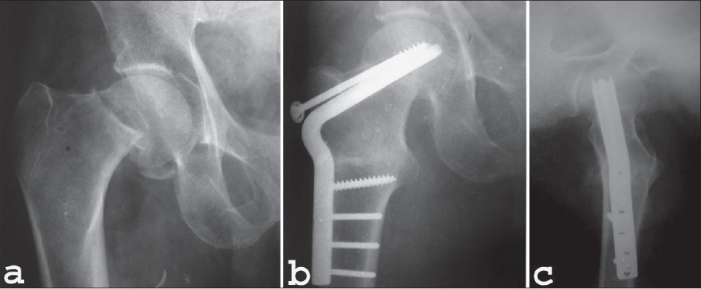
A: Antero-posterior radiograph (a) of 80 years male shows Garden stage IV and Pauwels type III femoral neck fracture. Anteroposterior radiograph (b) of the same patient after 2 years of modified Pauwels’ intertrochanteric osteotomy, shows sound union of fracture and of osteotomy. Femoral head is viable. Frog leg radiograph (c) of the patient at the 2 years of follow up
